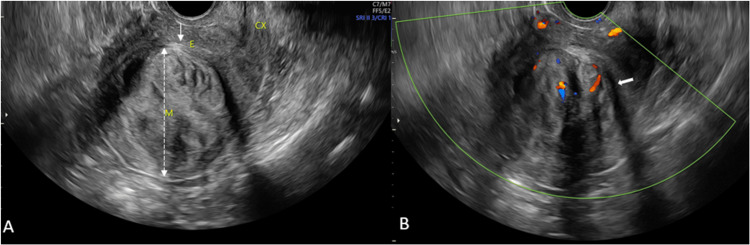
TVS ultrasonography B-mode (A) and color Doppler (B) longitudinal images of a 38-year-old female with complaints of abnormal uterine bleeding and dysmenorrhea A large uterine fibroid (M) is seen along the posterior myometrial wall, intramural in location, with endometrial contact (FIGO 3). It shows heterogeneous echotexture (long dashed double-headed arrow), uniform vascularity, and fan-shaped shadowing (solid arrow), displacing the endometrium (E) anteriorly (line arrow). CX, cervix; FIGO, International Federation of Gynecology and Obstetrics; TVS, transvaginal
